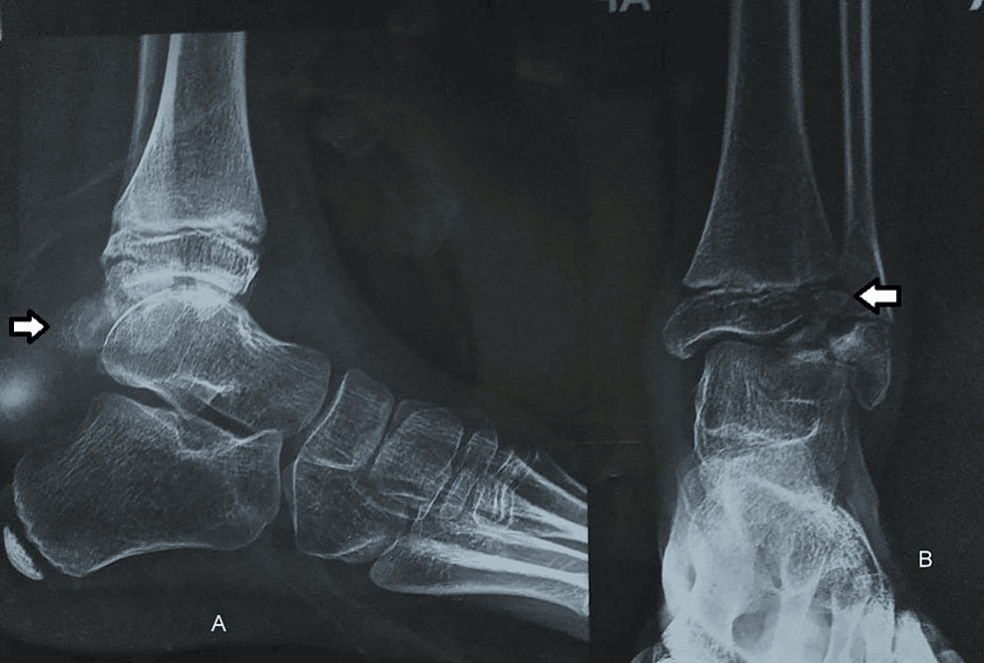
Preoperative lateral (A) and AP (B) radiographs of the ankle show bony outgrowths from the talus, which are directed posteriorly and laterally
